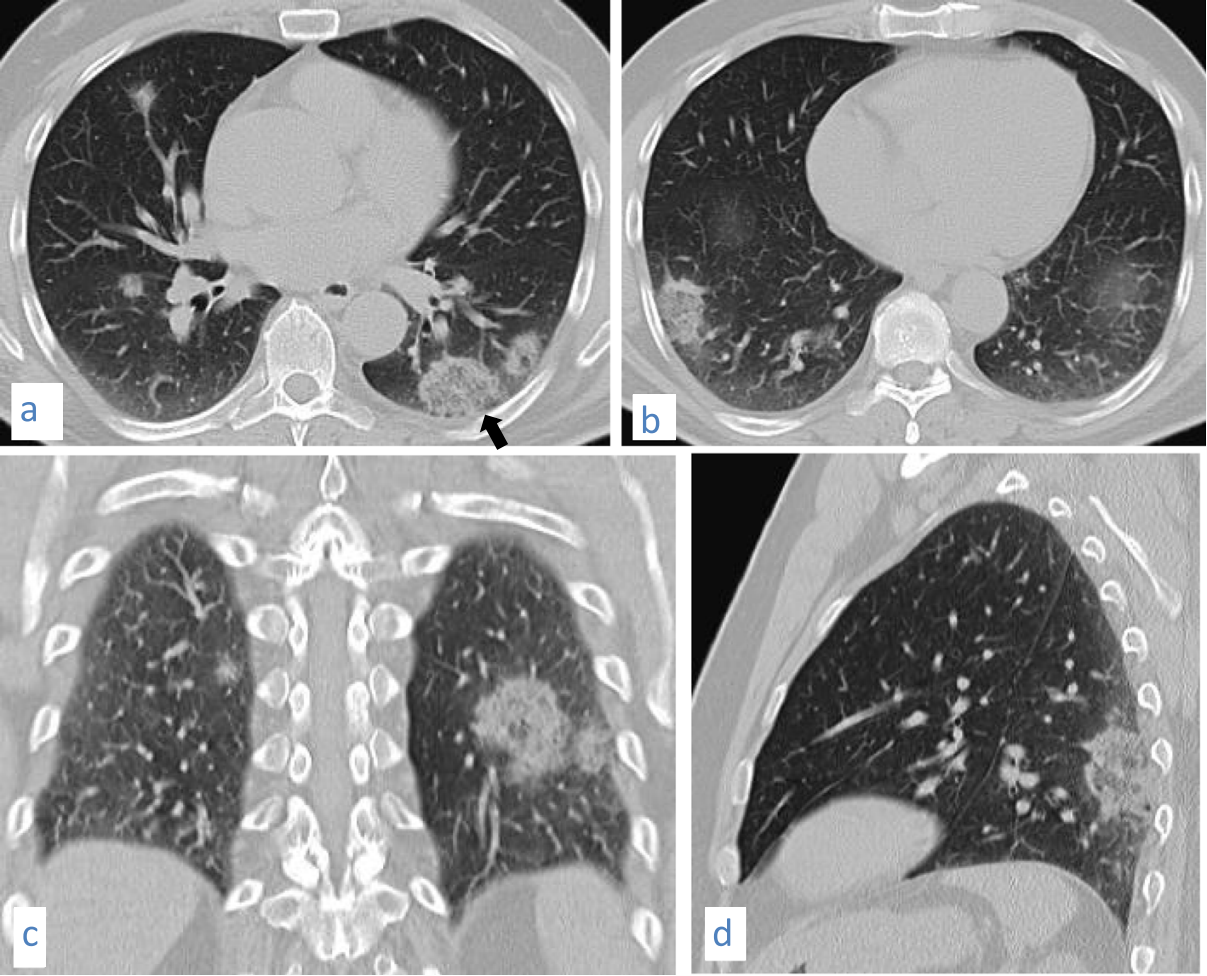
A 53-year-old male patient with COVID-19. CT chest axial images (a) and (b), coronal image (c), and sagittal image (d) show subpleural ground glass opacities with vascular thickening, nodules and masses some show “reversed halo” sign (arrow)
